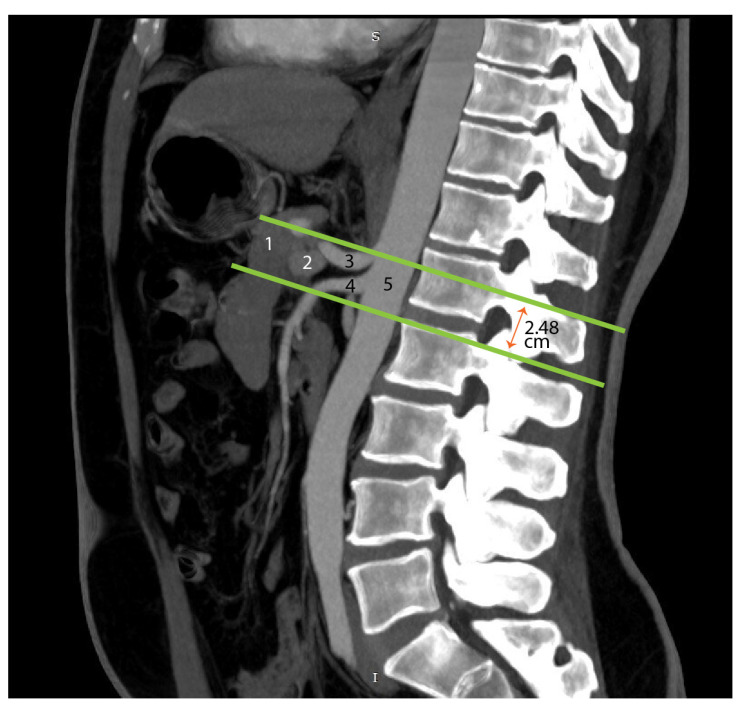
Computed tomography depiction of the mesopancreatic region. The green lines outline the boundary of the mesopancreas, a region identified as the retro-pancreatic retro-portal tissue. In the figure, the inferior boundary of the mesopancreas is 2.48 cm below the origin of the celiac trunk. 1—pancreas; 2—portal vein; 3—celiac trunk 4—superior mesenteric artery; 5—abdominal aorta.
